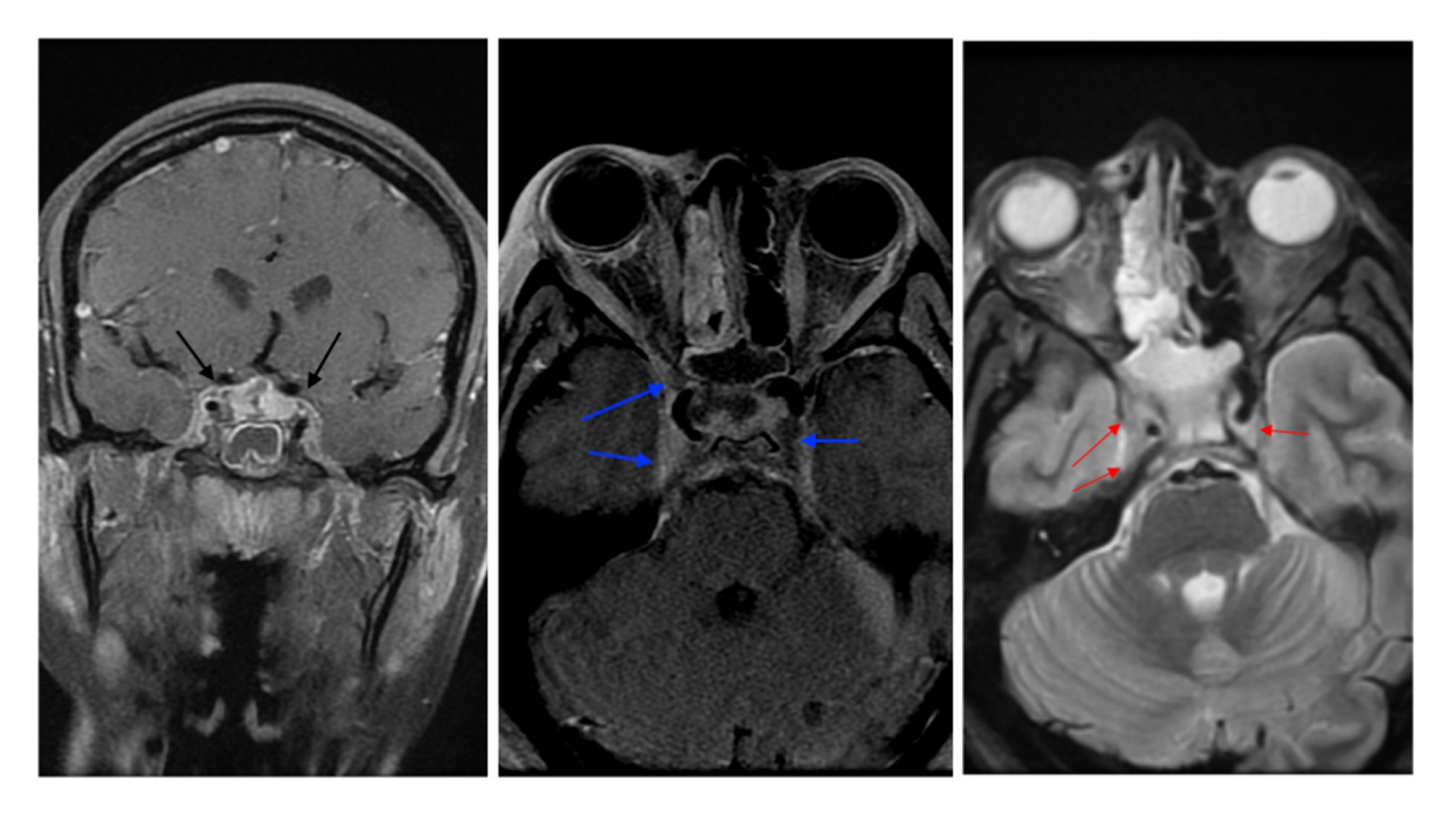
MRI showing filling defects indicating bilateral cavernous sinus thrombosis with the right side being greater than the left side (T1 contrast coronal: right, black arrows; T1 contrast axial: middle, blue arrows; T2 no contrast axial: left, red arrows).
